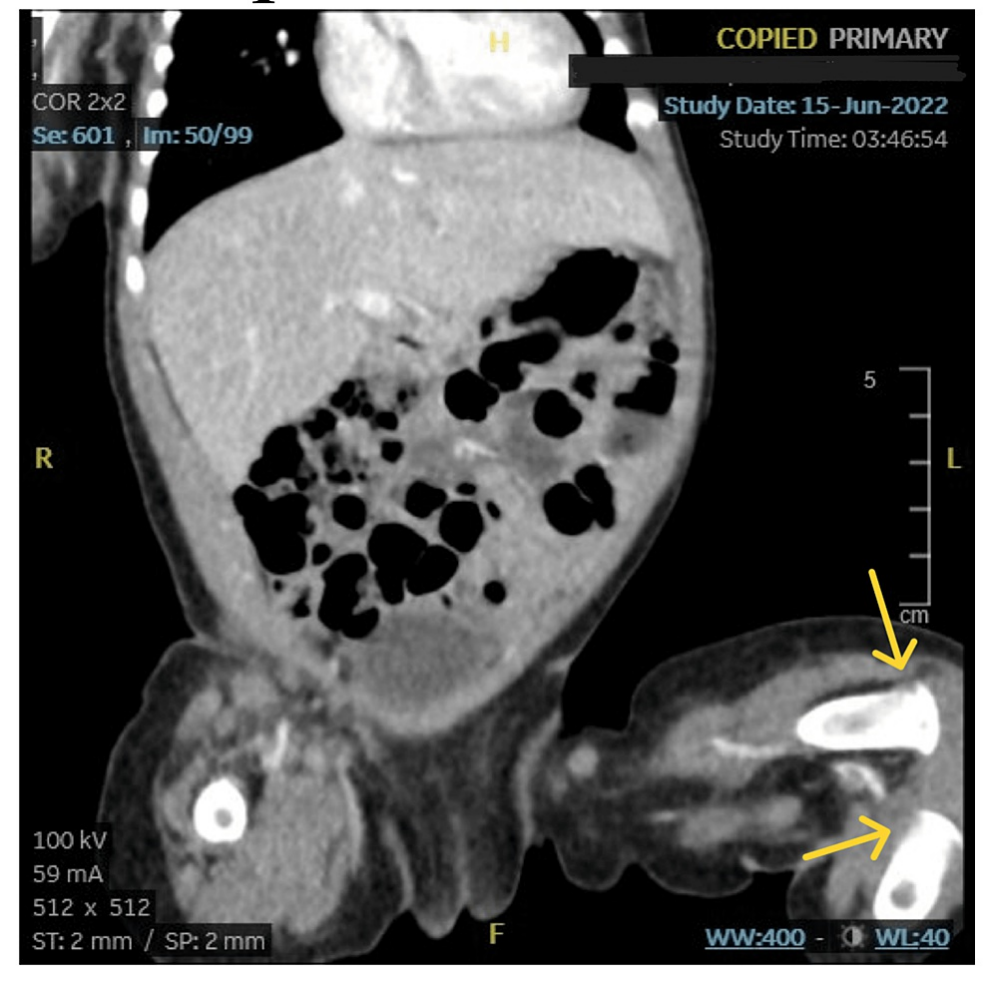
A CT scan of the abdomen revealed distal tibial and femoral fractures.
